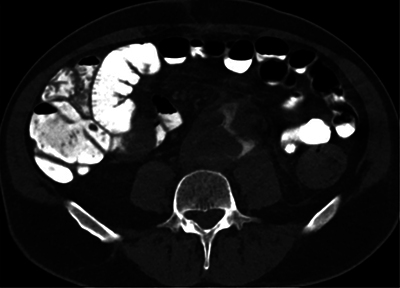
Axial CT scan showing leakage of digestive contrast into the aneurysm sac.
